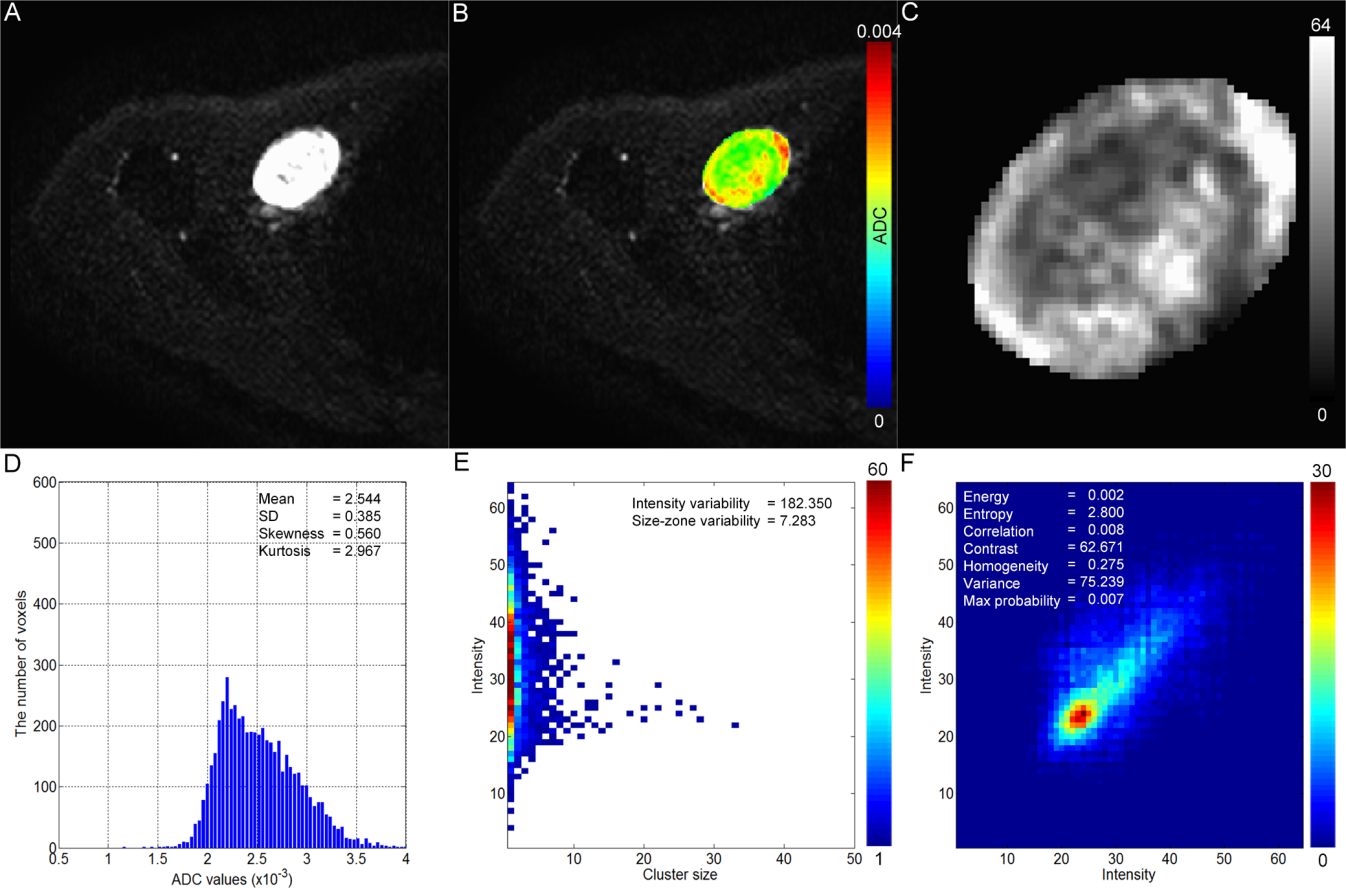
A case of benign myxoid-containing STT (schwannoma) in a 48-year-old female. DWI (A) shows a circumscribed high signal intensity mass in the right axillary region. Using a color ADC map (B), a discrete ADC map with 64 different values ranging from 1 to 64 (C), a histogram (D), a GLSZM (E), and a GLCM (F) were generated.
